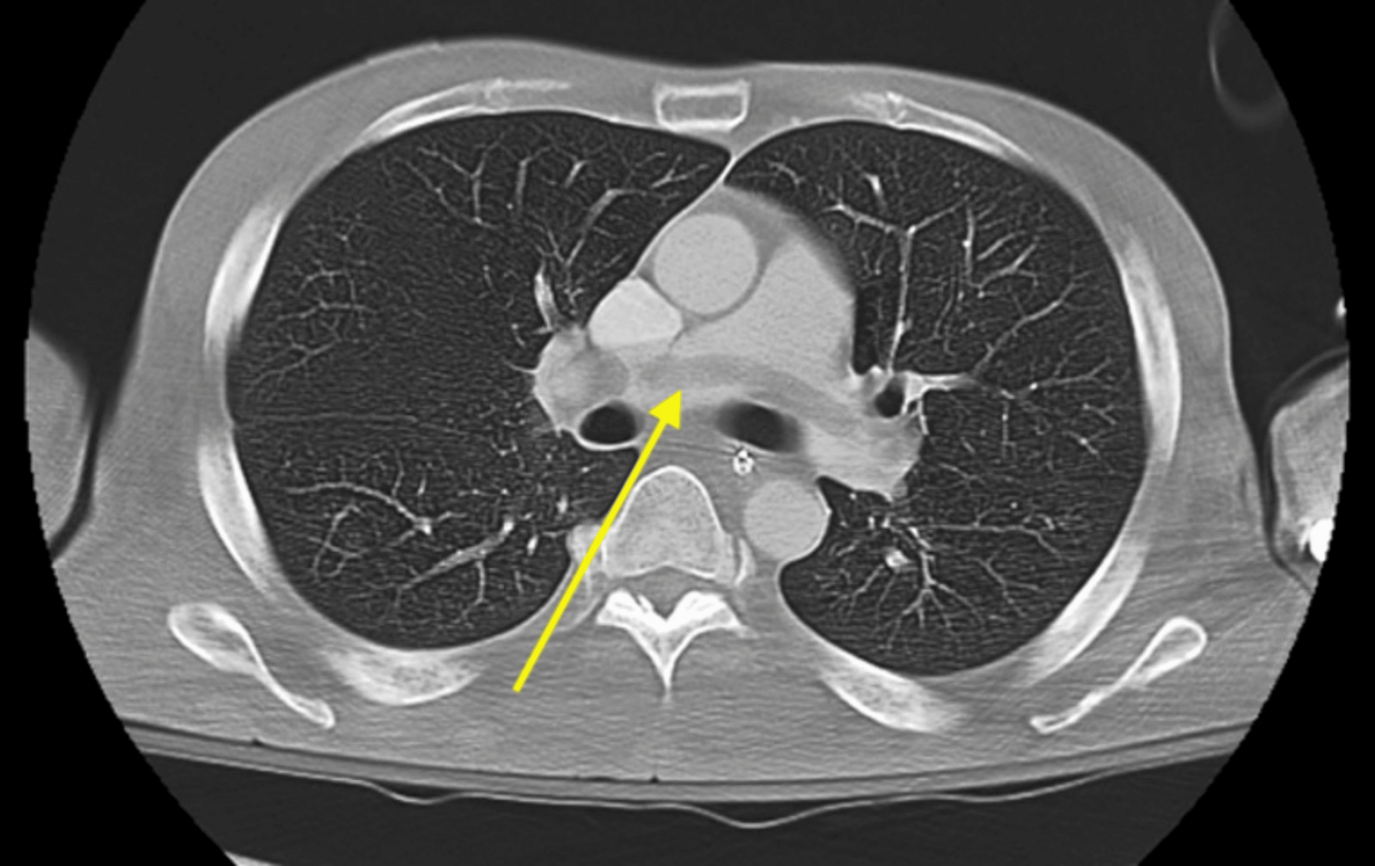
Massive saddle embolism seen on computed tomography pulmonary angiography.
